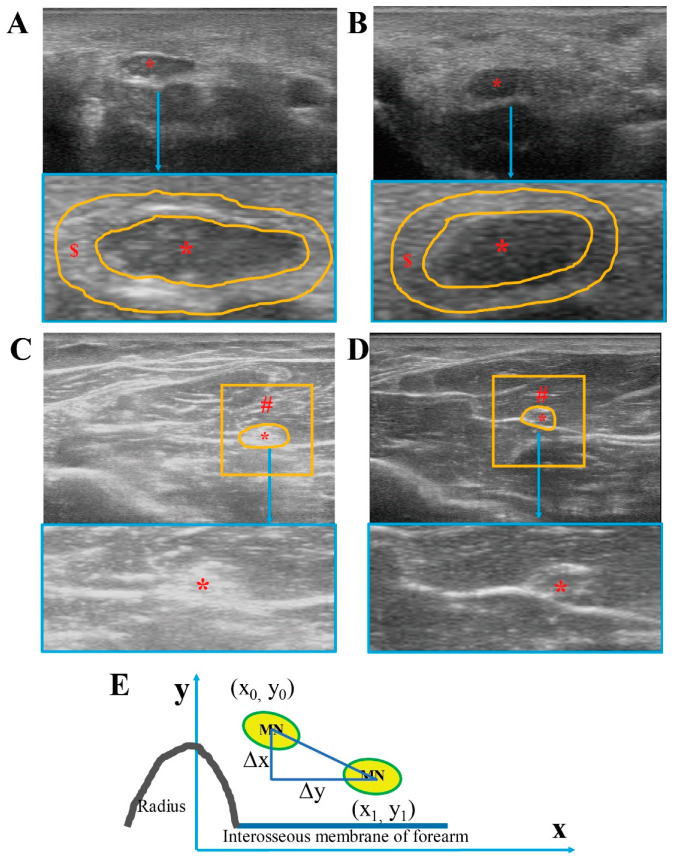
Ultrasound images of median nerve and surrounding tissue. Ultrasound images from CTS subjects at carpal tunnel (A) and at mid-forearm (C). Ultrasound images from healthy controls at carpal tunnel (B) and at mid-forearm (D). MN displacement was measured at mid-forearm as the difference between the centroid coordinates $\sqrt{\left({\Delta x}_{-}^{2}{\Delta y}^{2}\right)}$ (E). * = median nerve, $ = paraneural area (PA) indicated by the orange line in A, B, # = region of interest myofascial structure around the median nerve (1 cm^2^) except the median nerve and paraneural area.
